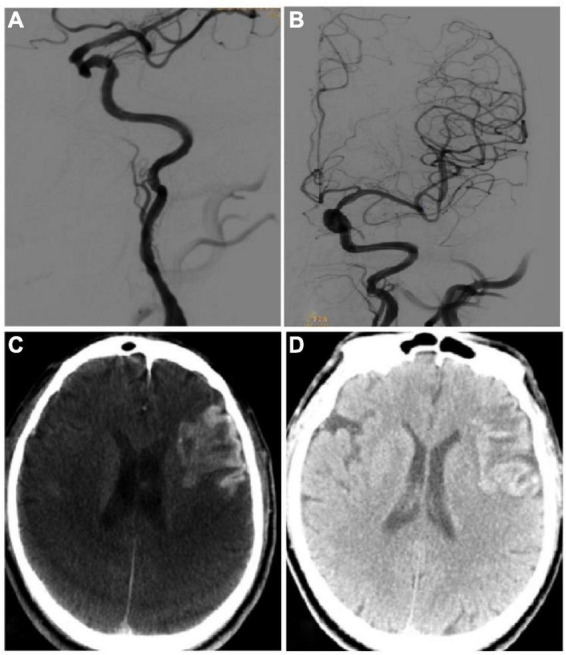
Imaging data of the same patient as in Figure 2. (A,B) On the 20th day after symptom onset, endovascular recanalization was performed to recanalize the long-segment occlusion of the left internal carotid artery, and two resolute stents (4.0 mm × 33 mm and 4.0 mm × 36 mm) were deployed in sequence. After recanalization, the occluded internal carotid artery was successfully recanalized. (C) Xper CT immediately after the surgery showed that the imaging classification of hemorrhagic transformation was type III. (D) Hemorrhage transformation was detected by CT plain scan on the next day after surgery.
